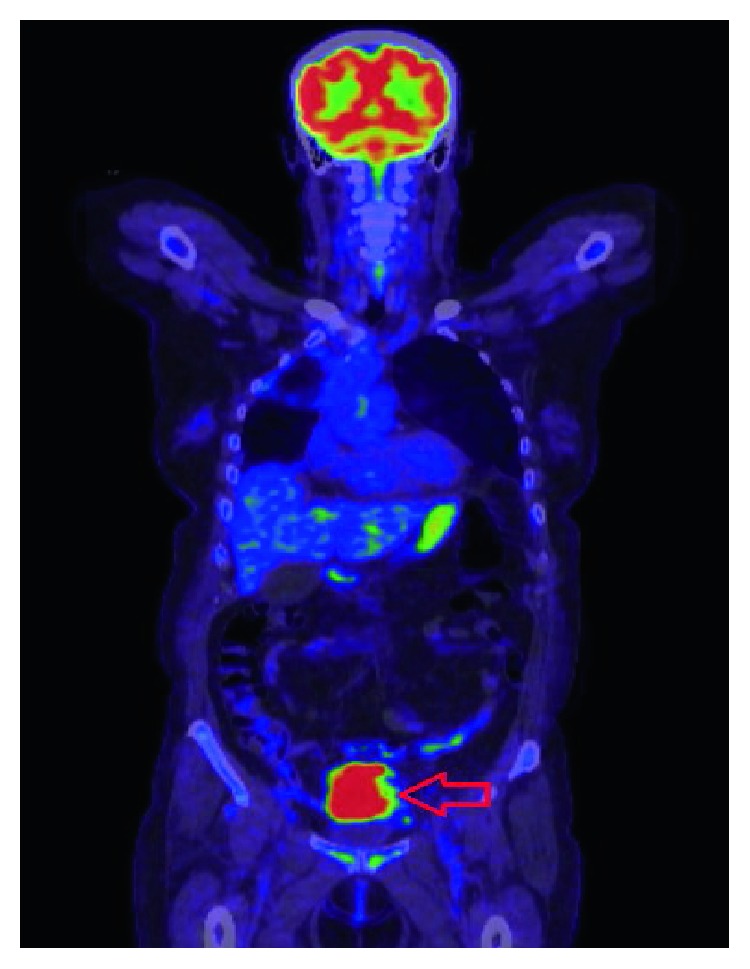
FDG-PET scan of our patient, following two weeks of serial radiological imaging, to monitor the foreign body. An intensely FDG-PET avid mass in the sigmoid colon was highlighted on imaging (arrow).
